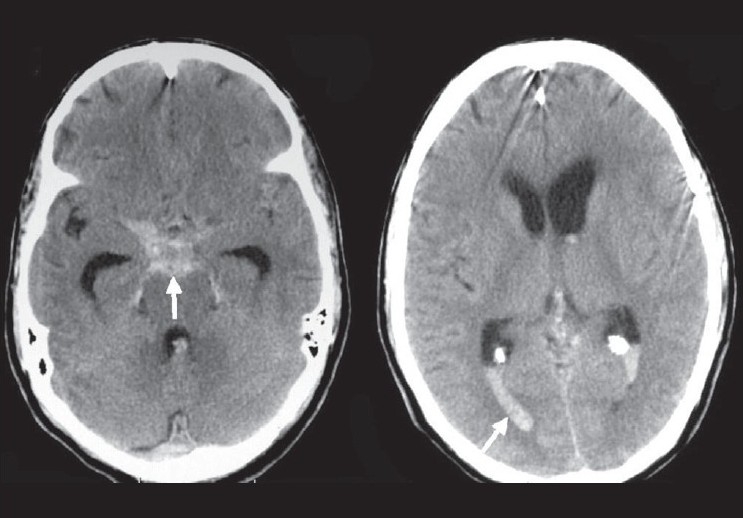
Computerized tomography (CT) shows diffuse subarachnoid hemorrhage (SAH) involving the basal cisterns, prepontine cistern (white arrow), left sylvian cistern, bilateral occipital horns (white arrow), third and fourth ventricle along with mild hydrocephalus
